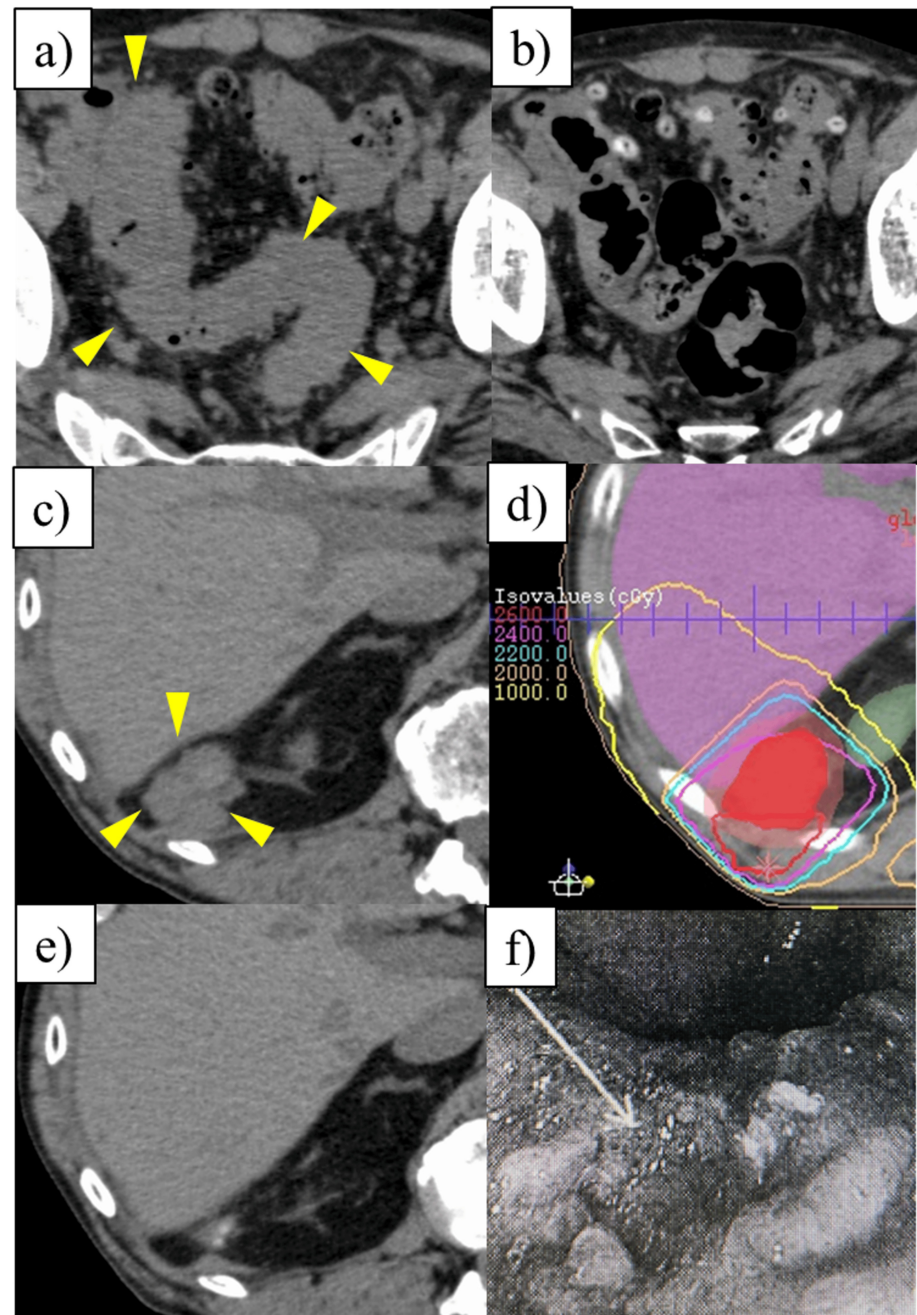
Course of the disseminated metastatic and recurrent disease. (a) CT image of pelvic dissemination before initiation of combined nivolumab and hyperthermia treatment. (b) CT image four months after the start of combined treatment with nivolumab and hyperthermia showing disappearance of pelvic dissemination. (c) CT image of the peritoneal dissemination on the dorsal side of the right kidney one month after the start of nivolumab and hyperthermia. (d) Dose distribution of radiotherapy for the peritoneal dissemination on the dorsal side of the right kidney (24 Gy/6 fractions), aiming to enhance the therapeutic efficacy of nivolumab. (e) CT images four months after completion of radiotherapy show metastatic disappearance. (f) Endoscopic photograph of the recurrent tumor in the remnant stomach.
